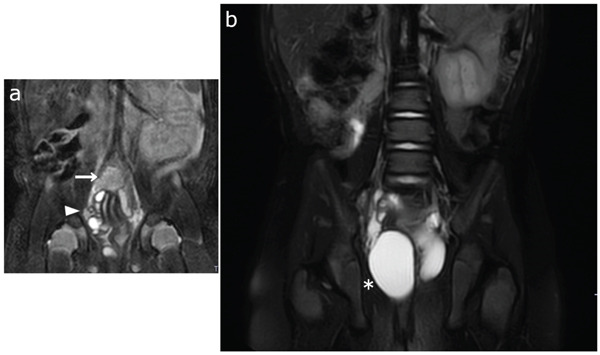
(a) Neonatal magnetic resonance imaging shows a hypoplastic right kidney (arrow) and a small tubular structure consistent with a ureter (arrowhead). (b) During follow‐up, the kidney gradually became indistinct; however, progressive dilation of the right obstructed hemivagina was observed at 1 year and 8 months of age (asterisk). Based on these findings, obstructed hemivagina and ipsilateral renal anomaly syndrome was diagnosed.
